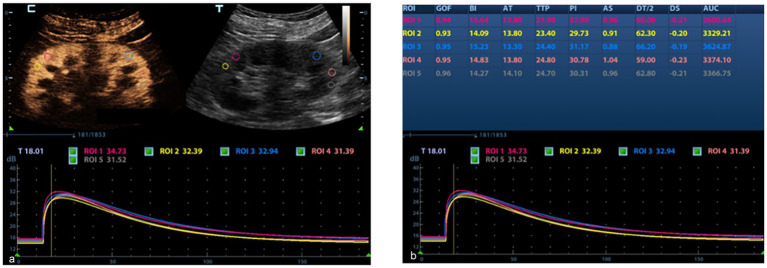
TIC of a forward flow insufficiency renal contrast enhancement. (a) Cortical sampling site and corresponding TIC. The screen displays the contrast-enhanced image (C) alongside the conventional ultrasound image (T). (b) Plot of ROIs analyzed by dedicated software, with fitted curves generated for each ROI (lower panel). The patient was a 64-year-old male diagnosed with intestinal obstruction and shock. CEUS parameters were characterized by prolonged AT and TTP,and a decreased AUC. This perfusion pattern may reflect delayed cortical microvascular inflow and reduced tissue perfusion, potentially suggesting hemodynamic hypoperfusion requiring early circulatory optimization.
